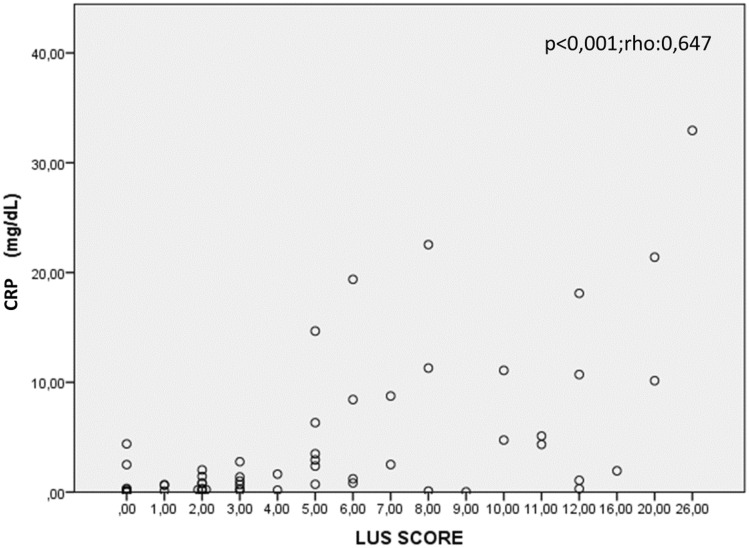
Correlation between lung ultrasound score (LUS) and C-reactive protein (CRP) levels
